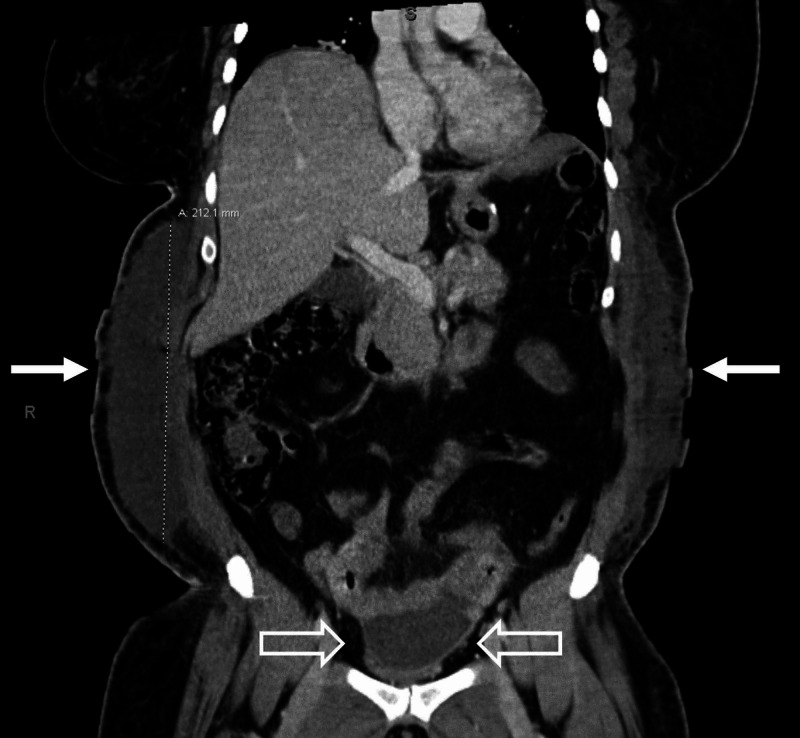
Coronal CT image showing bilateral flank fluid collections (white arrows) and an intraperitoneal fluid collection (blank arrows).
